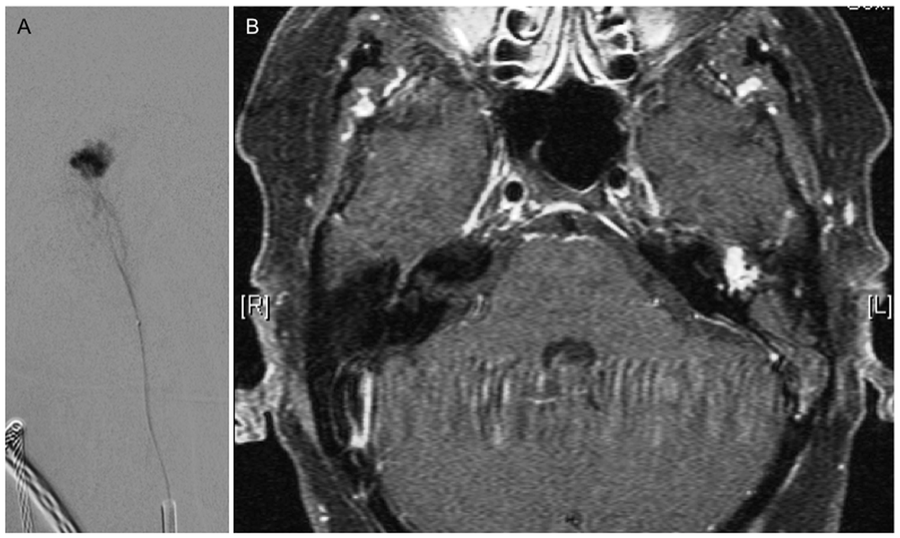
42 year old patient with left sided PT for one year. A: Superselective angiographic microcatheter injection before preoperative embolization with particles. B: MRI ce-T1 fs shows a Glomus tympanicum paraganglioma: enhancing mass in loco typico.
